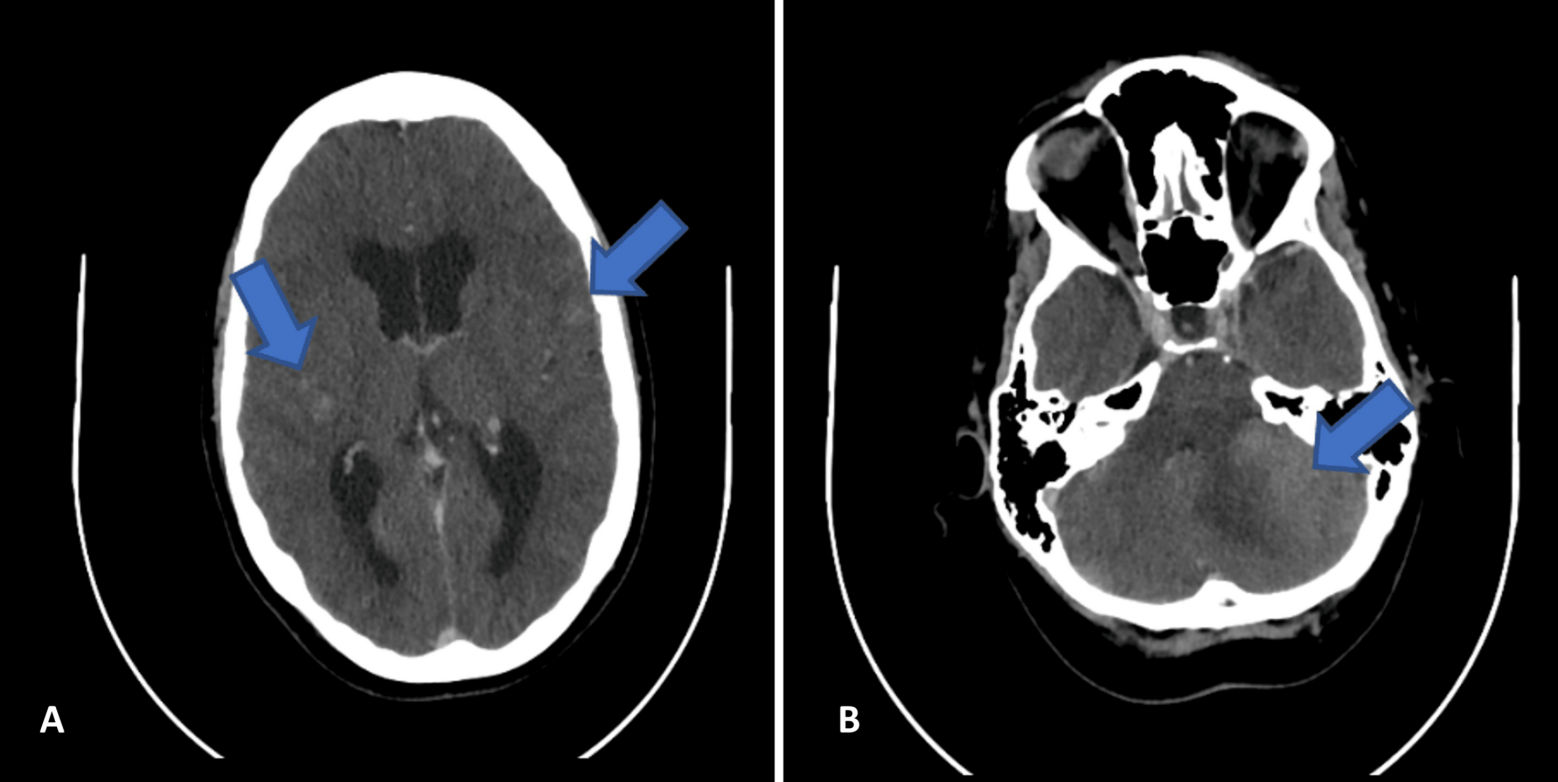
Brain CT scan in the emergency department. Blue arrows showing infiltrative (A, B) brain lesions. Abbreviation: CT, computed tomography.
